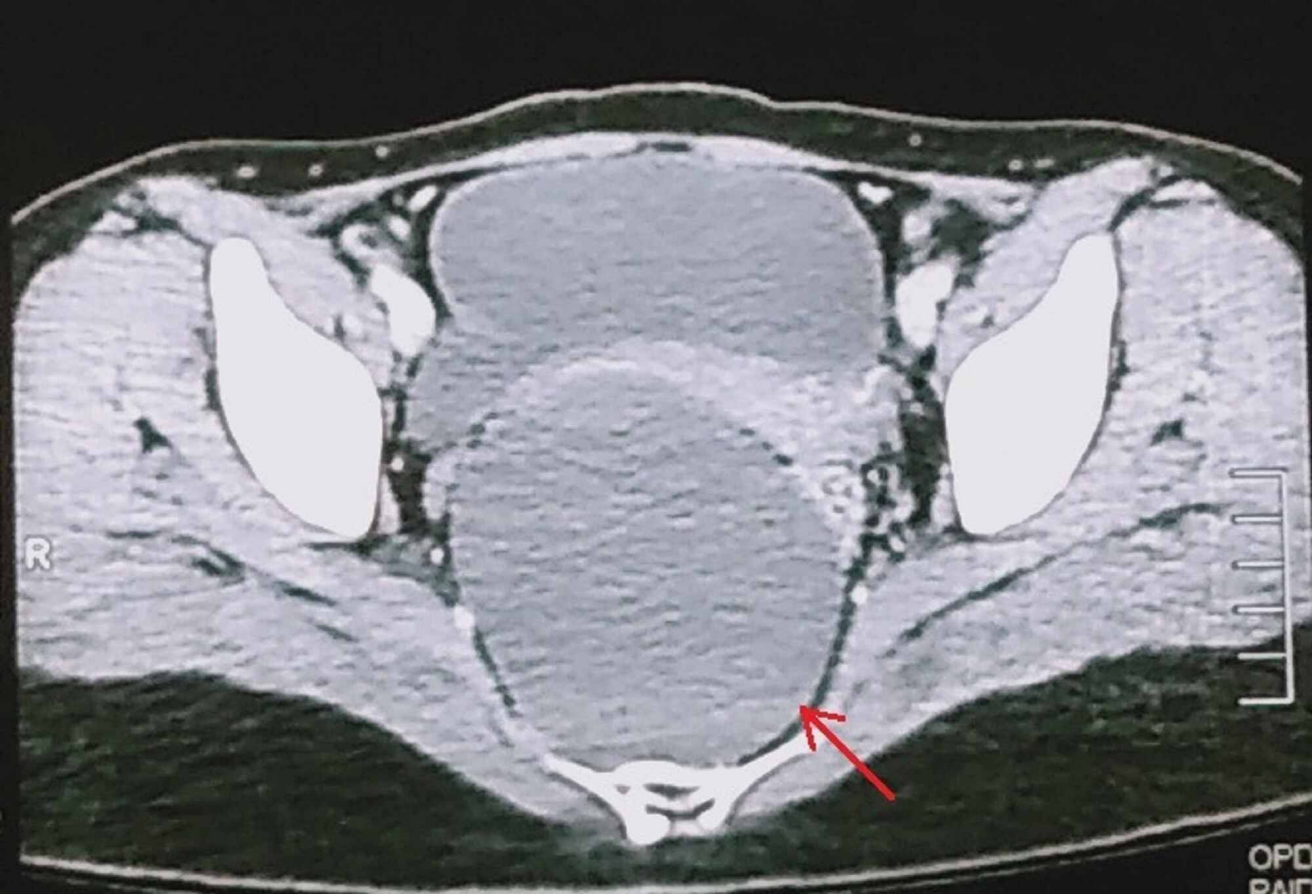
CT scan showing a well-defined cystic area anterior to the sacrum, displacing the rectum towards the left CT, computed tomography
